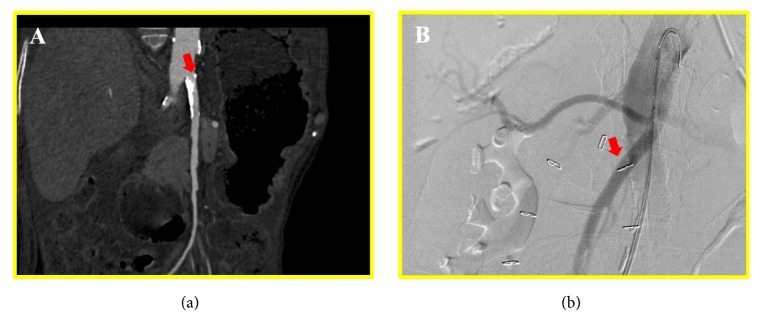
CT angiography abdomen revealed stenosis of mesenteric artery (a). Arteriogram indicated patent mesenteric artery (b). Arrow: mesenteric artery.
